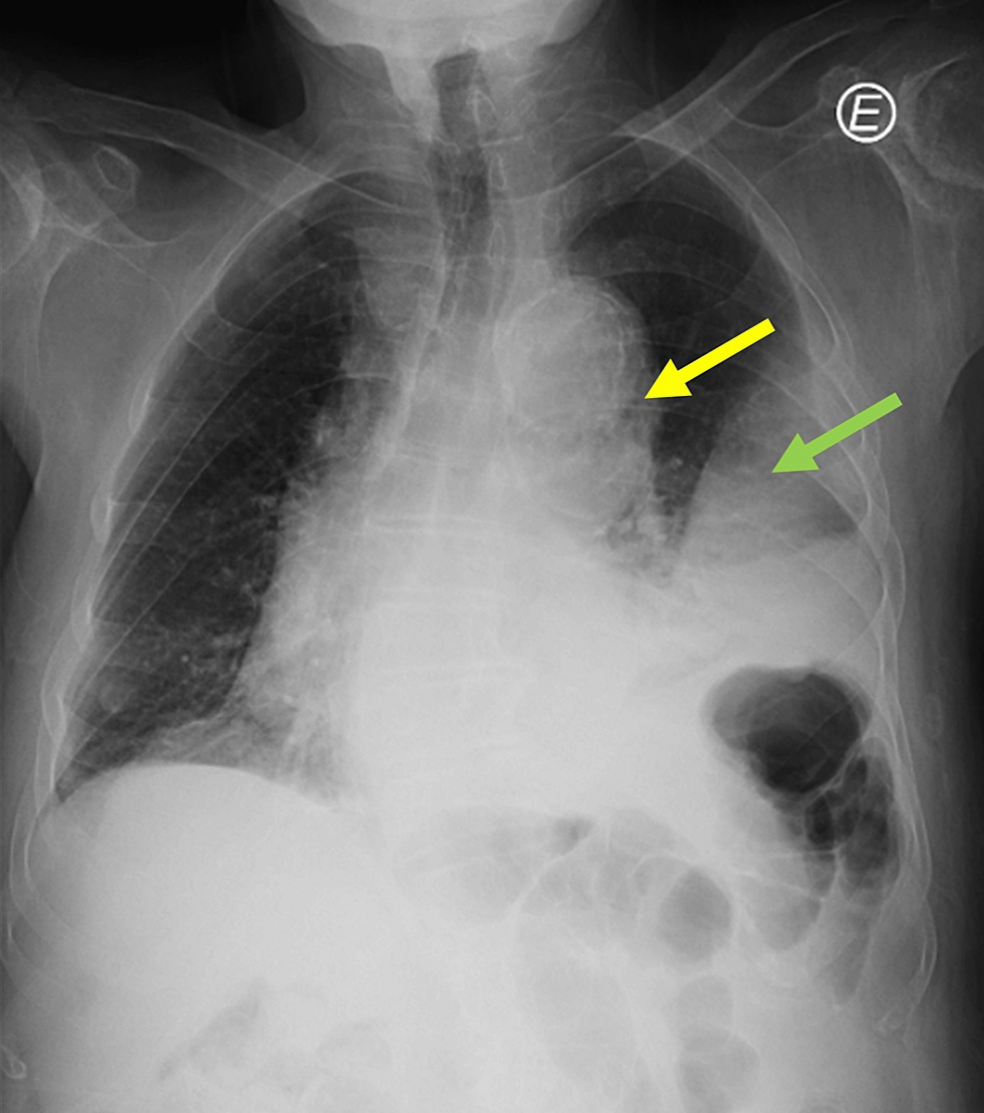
Chest X-ray with periaortic small air bubbles (pneumomediastinum) marked by the yellow arrow, atelectasis, and left pleural effusion (green arrow)
